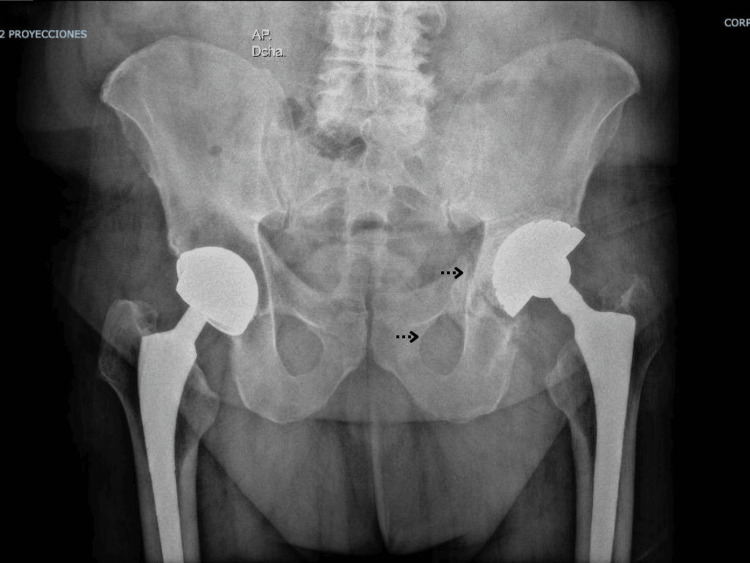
Preoperative AP pelvic radiograph showing pelvic discontinuity with medial migration of the inferior hemipelvis and asymmetry of the obturator ring.
